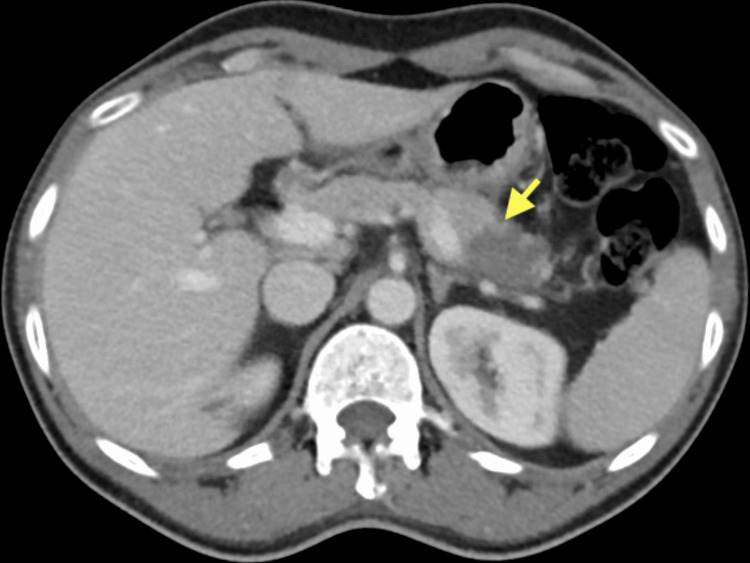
CT of the abdomen. CT scan demonstrating the interval reduction in the pancreatic lesion (yellow arrow).
